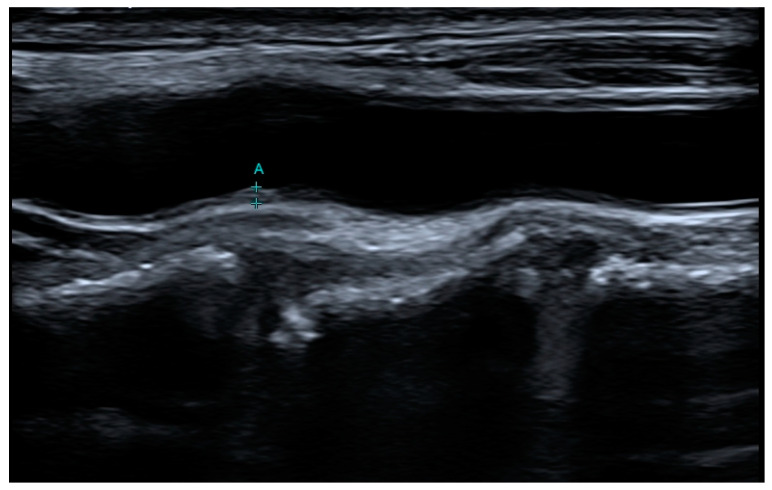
Example of measurement of intima media thickness (IMT) in ultrasound examination of the carotid arteries. A—IMT measurement, + measurement markers.
